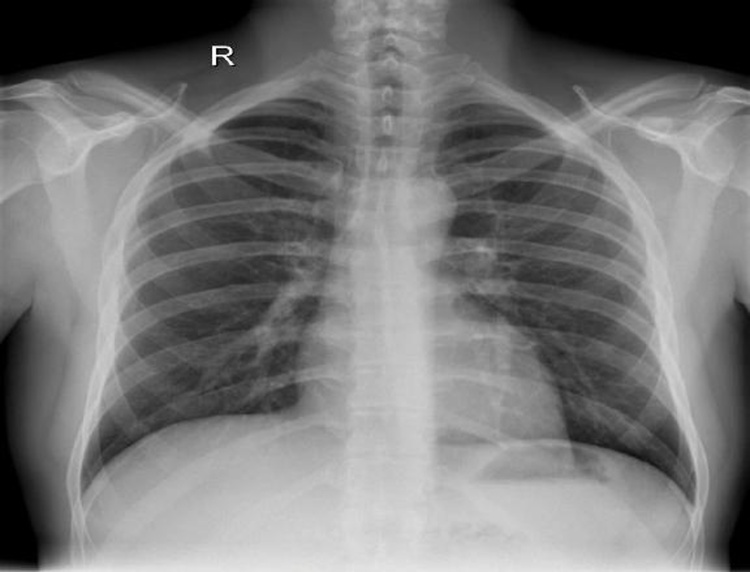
Chest X-Ray without pathological findings 3 months later.
